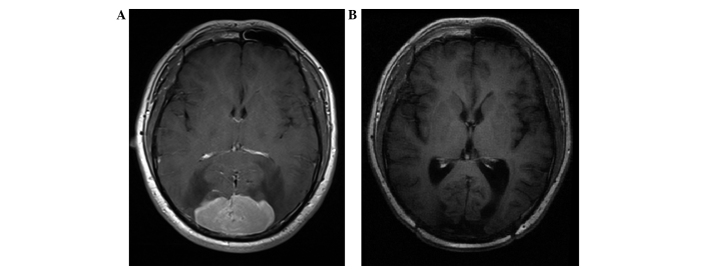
Magnetic resonance imaging revealing the intracranial myeloid sarcoma (A) prior to and (B) subsequent to the combined therapy.
